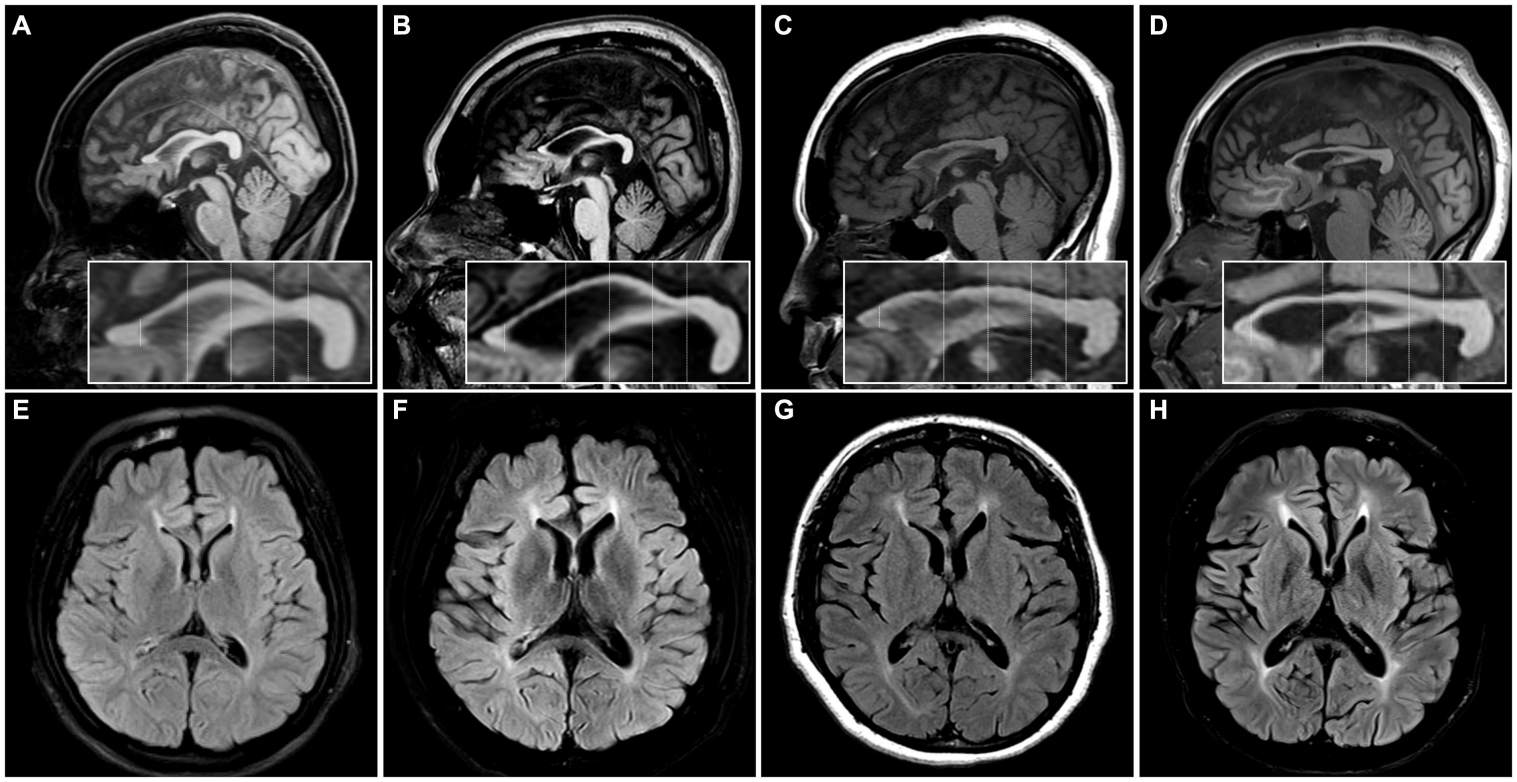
The initial and follow-up images of two patients. (A–D) MR images of SN-1. (A,B) The focal thinning of region 3 in the initial image was further thinned and other regions of the corpus callosum were also slenderized on the MRI performed 12 years later. (C,D) The forceps minor region signal abnormality became more distinct in the subsequent image, and the forceps major region signal abnormality with ventricular widening was observed in the follow-up image. (E–H) MR images of the SN-4. (E,F) The thinning in the anterior half of the corpus callosum was still observed on the MRI performed 3 years later, although there was no significant change. (G,H) The forceps minor and major regions signal abnormalities with ventricular widening were more distinct in the follow-up image.
